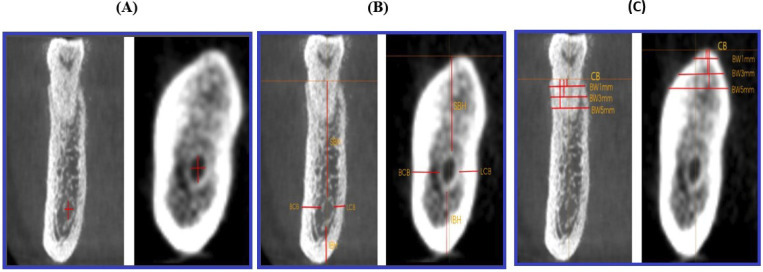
Measurements of maximum vertical and horizontal diameters of the mandibular canal (MC) in dentate and edentulous sides (A); Measurements of superior bone height (SBH), inferior bone height (IBH), buccal cortical bone (BCB) and lingual cortical bone (LCB) on the dentulous and edentulous sides (B); and bone width measurements at three heights (1mm, 3mm, and 5mm), the reference lines at the three points (the vertical lines) below the coronal point of alveolar bone (CB) and the radiographic measurements (the horizontal lines) from buccal to lingual margins on the dentulous and edentulous sides (C).
